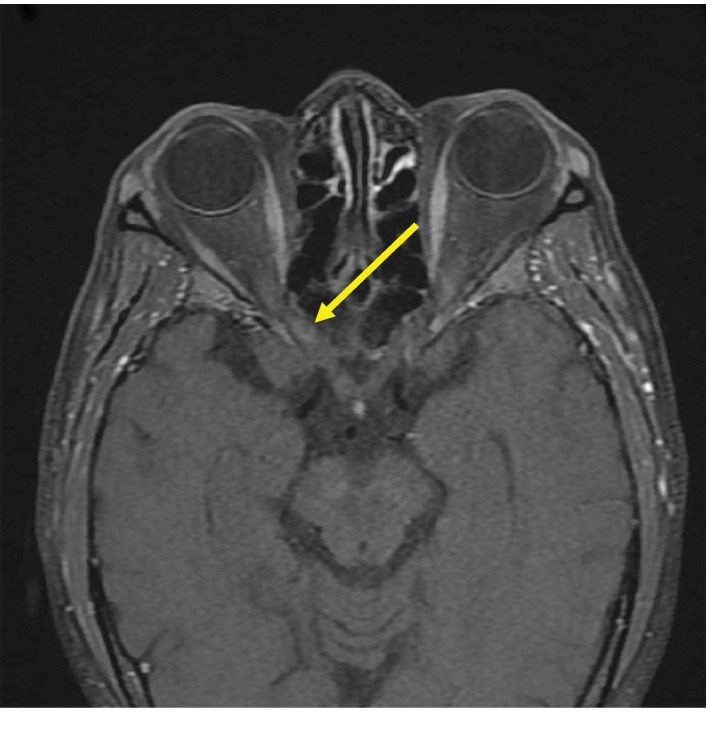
MRI of the brain and orbits with contrast showing lymphoma infiltration in the right optic nerve.
